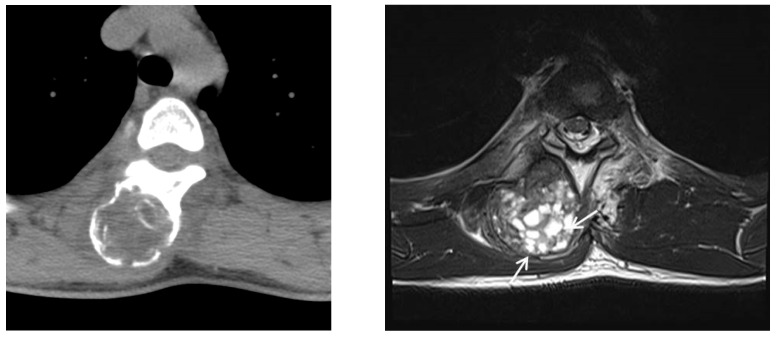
(Left) On follow-up CT, the paraspinal mass had enlarged compared with previous CT images. Extrinsic erosion to adjacent bone was also noted. (Right) Follow-up MR imaging demonstrates that multiple small cystic lesions with fluid–fluid levels (arrows) had developed in the mass, which suggested aneurysmal bone cystic change.
